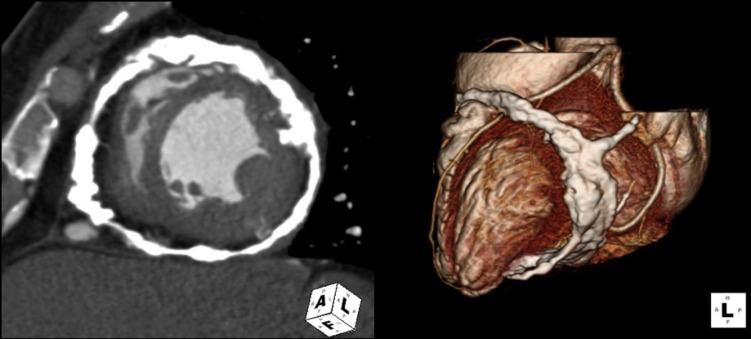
Multi-detector CT demonstrated heavy eggshell calcification encircling the heart.
